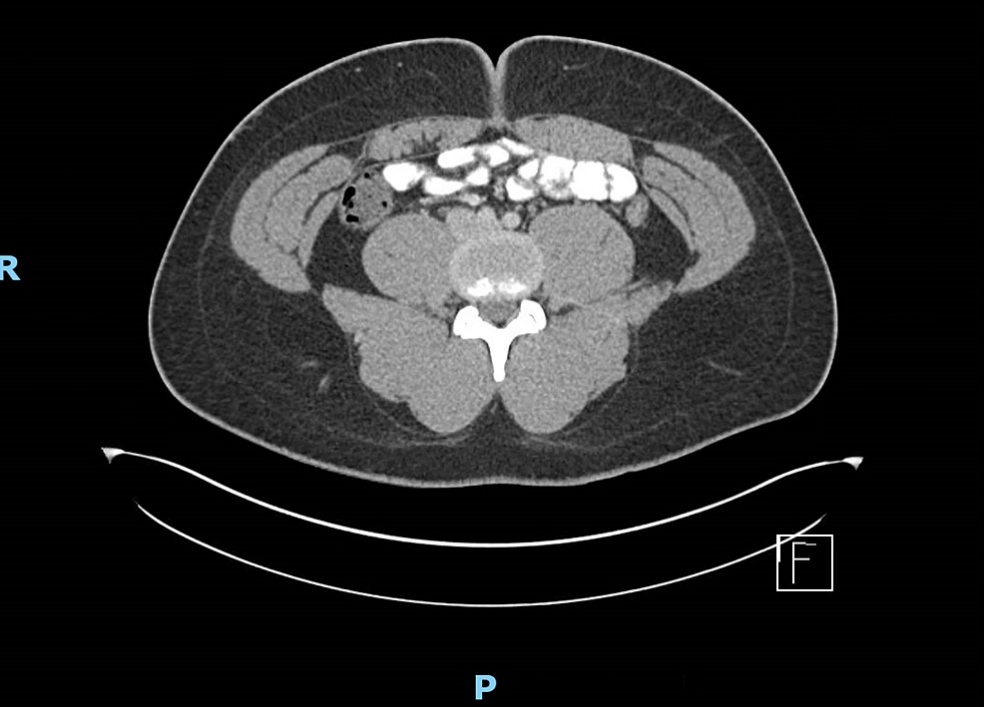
Computed tomography of the abdomen and pelvis did not show evidence of any extracolonic manifestations
